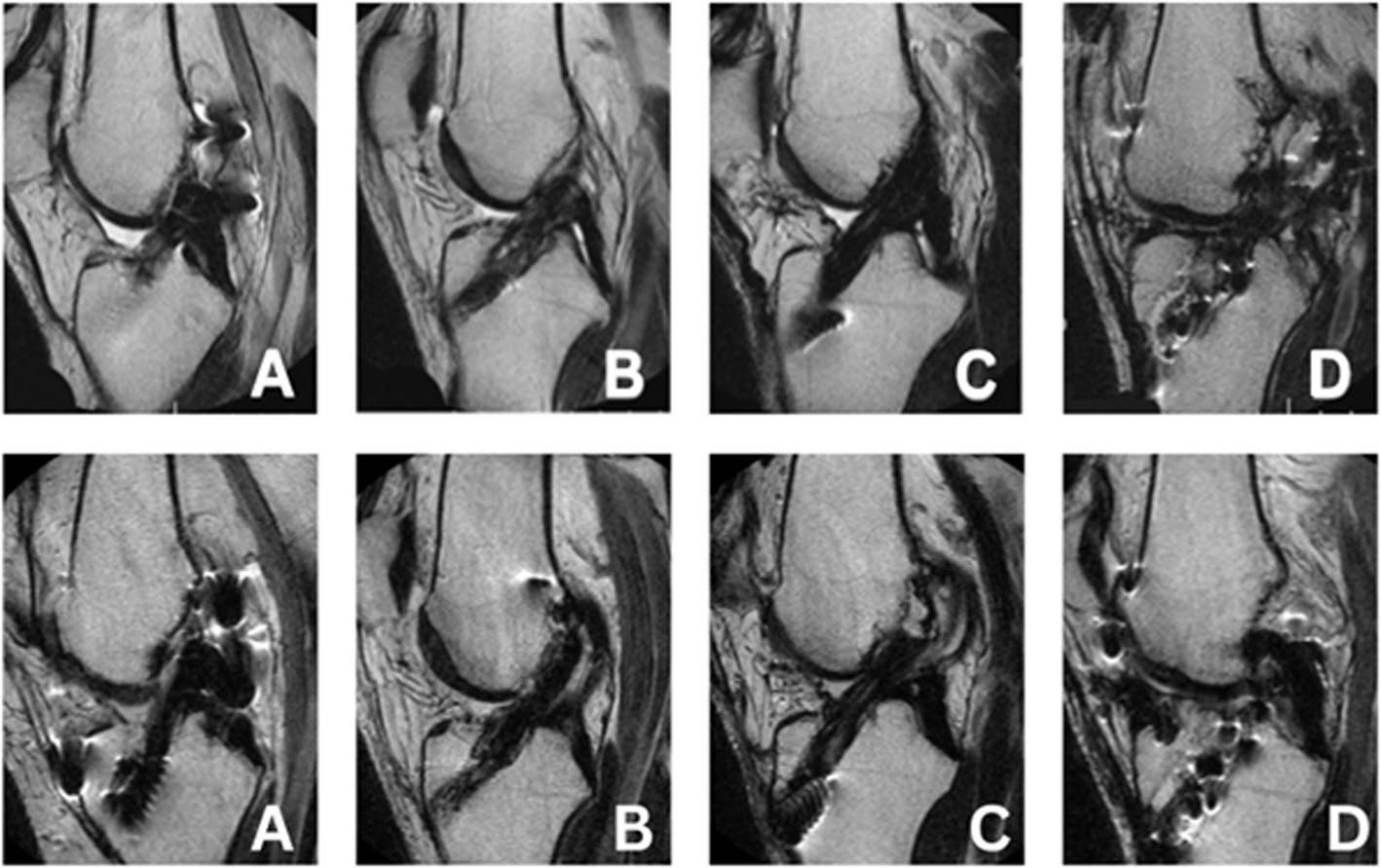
12-year (top row) and 20-year (bottom row) MRI Images of Z-Lig™ Reconstructions, FSE T-2 weighted in the sagittal plane: A) Subject P-01; B) Subject P-03; C) Subject P-04; and D) Subject P-07
